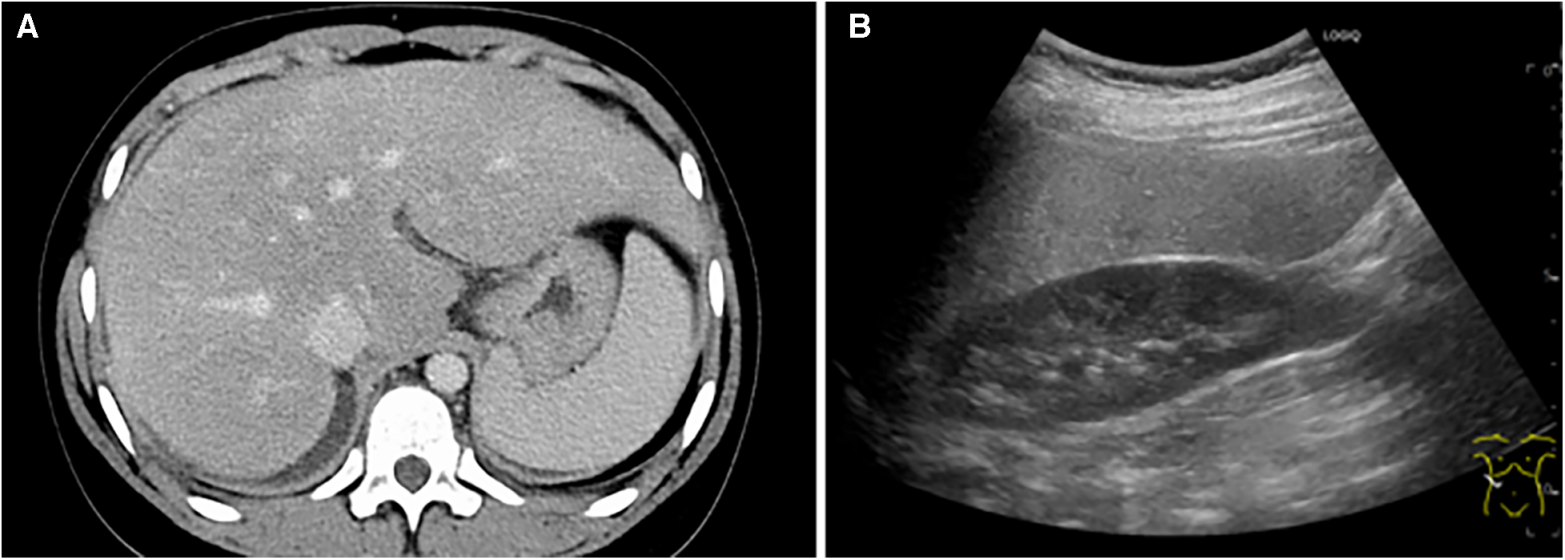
Other imaging data obtained after the onset of severe acute respiratory syndrome coronavirus 2 infection. (A) A contrast-enhanced abdominal computed tomography scan showing hepatomegaly and splenomegaly. (B) An abdominal echocardiographic image showing increased echoluminance of the liver, which indicates a fatty liver.
